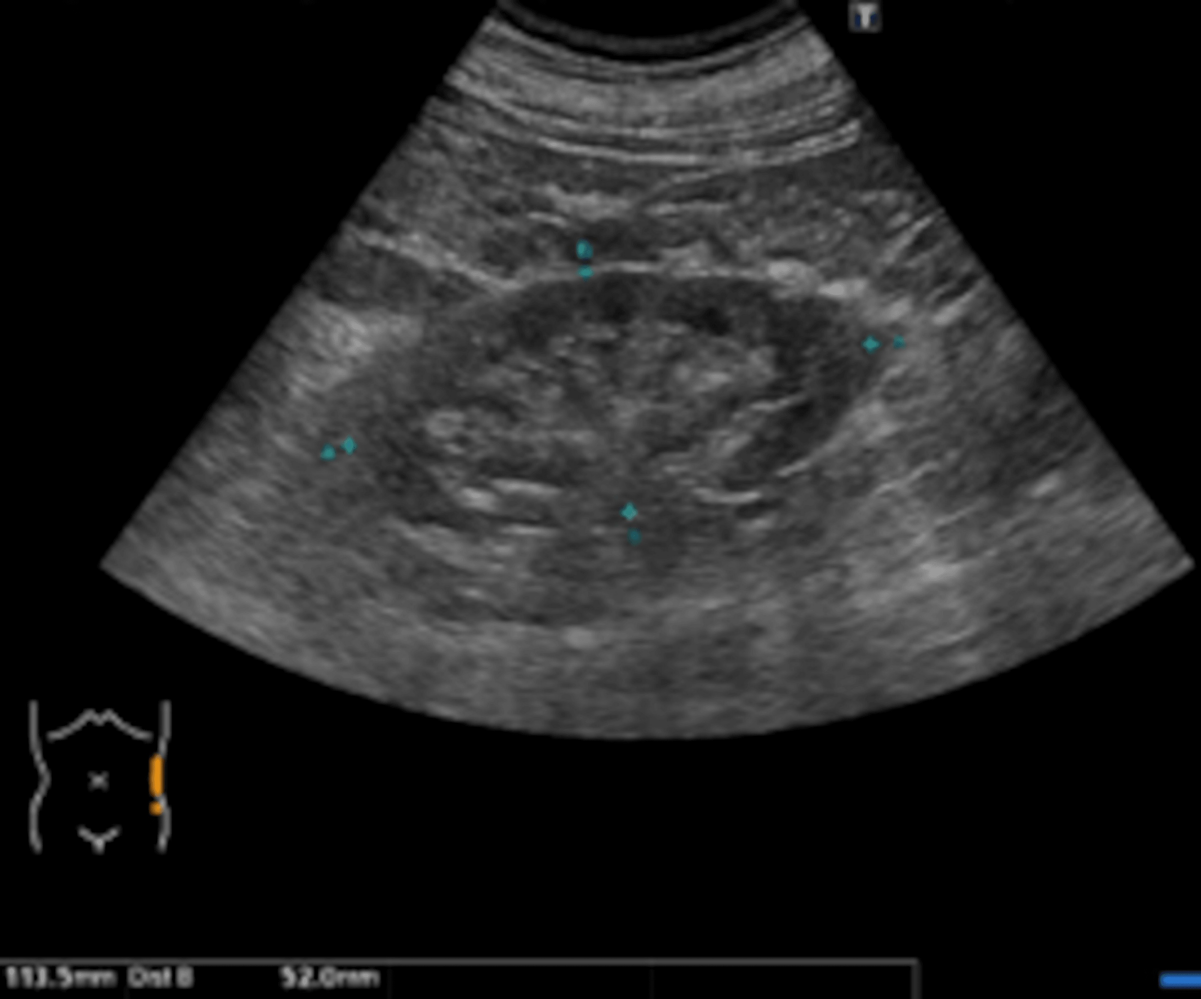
Left renal ultrasound findings. Renal ultrasound demonstrates irregular morphology with mild atrophy of the left kidney, which measured 113.5 mm in length and 52 mm in width, with no evidence of hydronephrosis.
